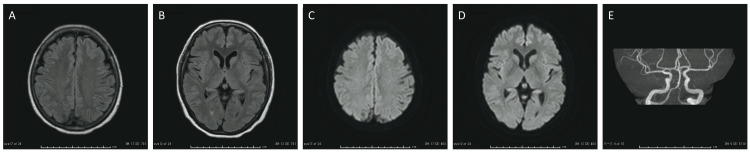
Brain MRI of Case 3 Fluid-attenuated inversion recovery (FLAIR) images (A, B) and diffusion-weighted images (C, D) of the brain MRI showed no old or acute lesions. A magnetic resonance angiography image (E) showed no abnormal findings that were consistent with vasculitis.
